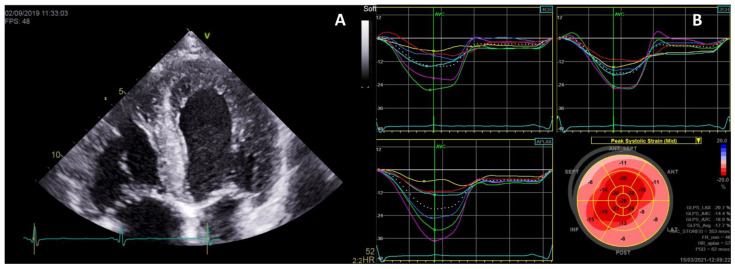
Apical sparing pattern of global longitudinal strain (GLS) in a Fabry patient. Transthoracic echocardiogram of a 48-year-old male Fabry patient showing symmetrical LVH in a four-chamber view (A) and 2 D-strain analysis by speckle tracking revealing an apical sparing pattern of GLS (B).
